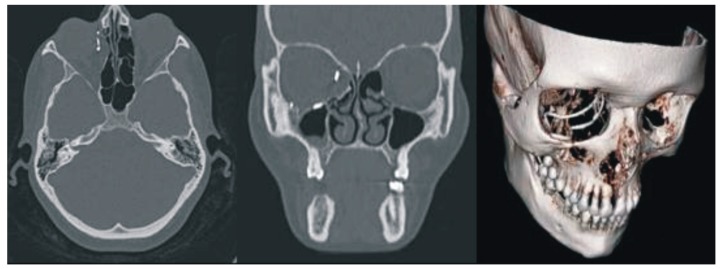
Facial CT images with 3D reconstruction at one month after surgery.
